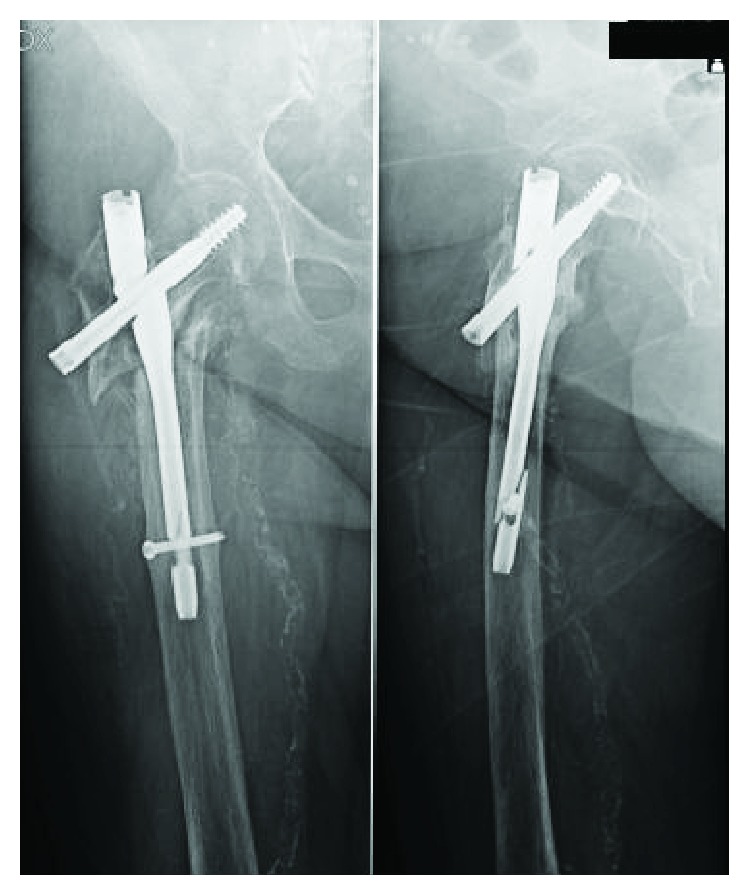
After 3 years, the X-rays (done in Emergency Room) showed the breakage of the nail in its proximal part and distal part.
